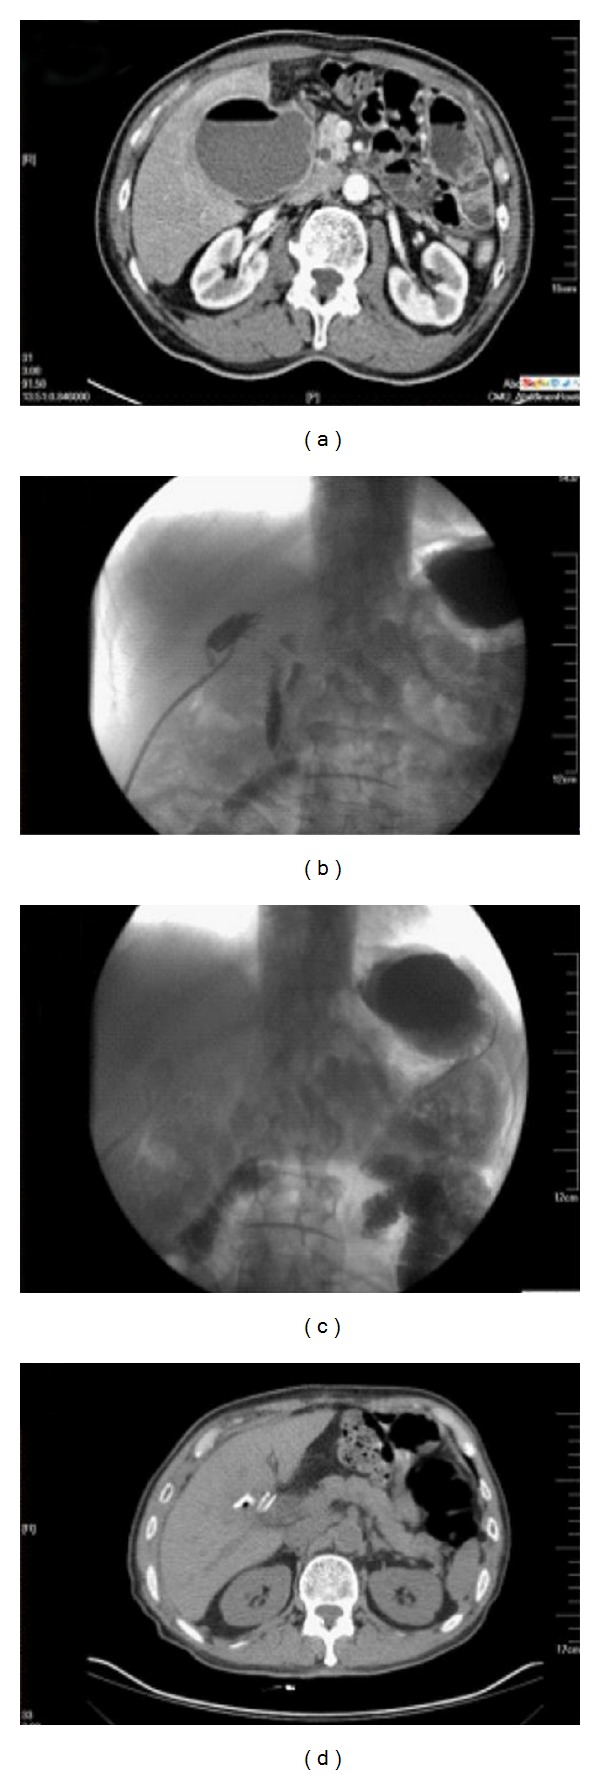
(a) A cyst-mass in the area of hepatic portal pneumonia was seen through abdominal CT. (b) When contrast agent was injected from the catheter, it was found to flow into the duodenal stump on the image. (c) When gastrointestinal X-ray was performed, contrast agent in stomach flow into jejunum directly. (d) Abdominal CT demonstrated that the cyst-mass in the area of hepatic portal disappeared.
